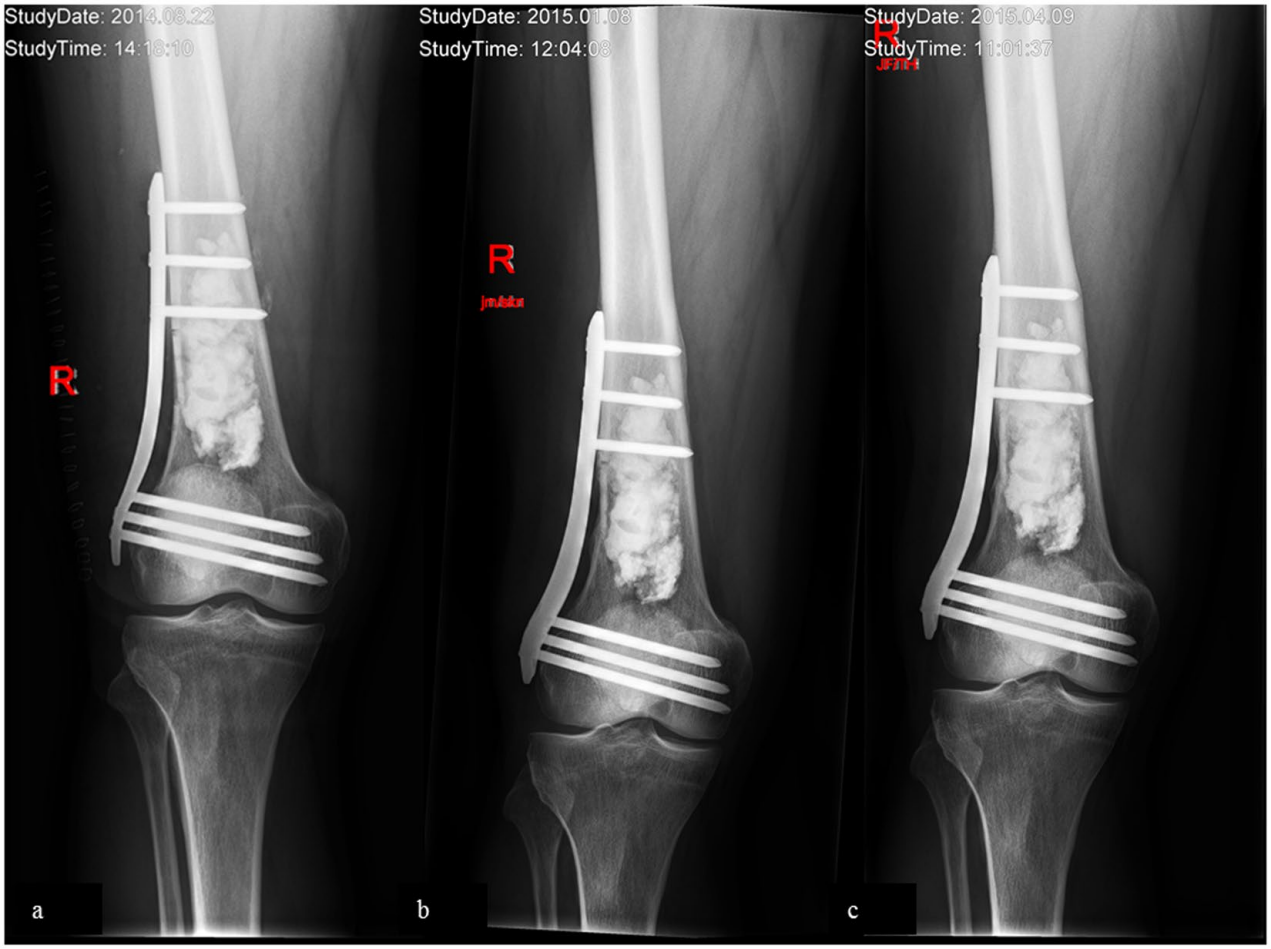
(a–c) X-ray of a 64 year-old male patient few days following curettage of an enchondroma of the right distal femur. To enhance primary stability, a plate osteosynthesis was done. (b and c) Follow-up x-rays 5 and 11 months following index surgery showing integration but no resorption of the bone graft substitute.
